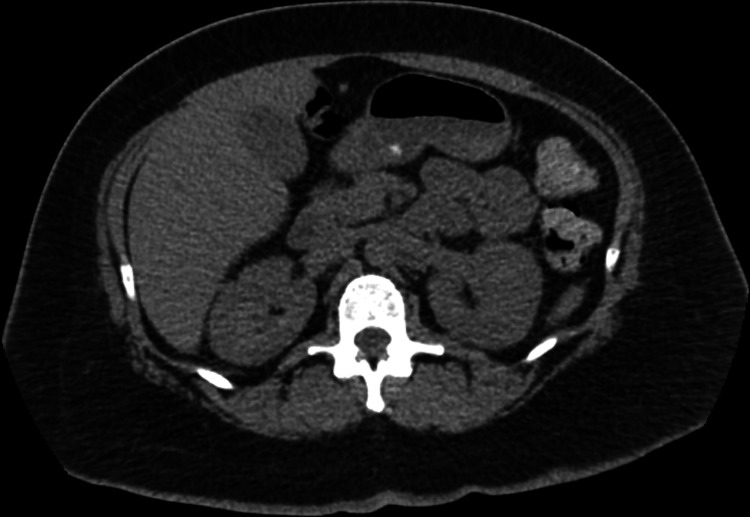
Abdomino-pelvic CT scan 1 Axial abdominopelvic CT scan at the level of the lung bases. The scan shows no evidence of bowel obstruction, perforation, or abscess. The appendix is intact, and no abnormalities are noted.
